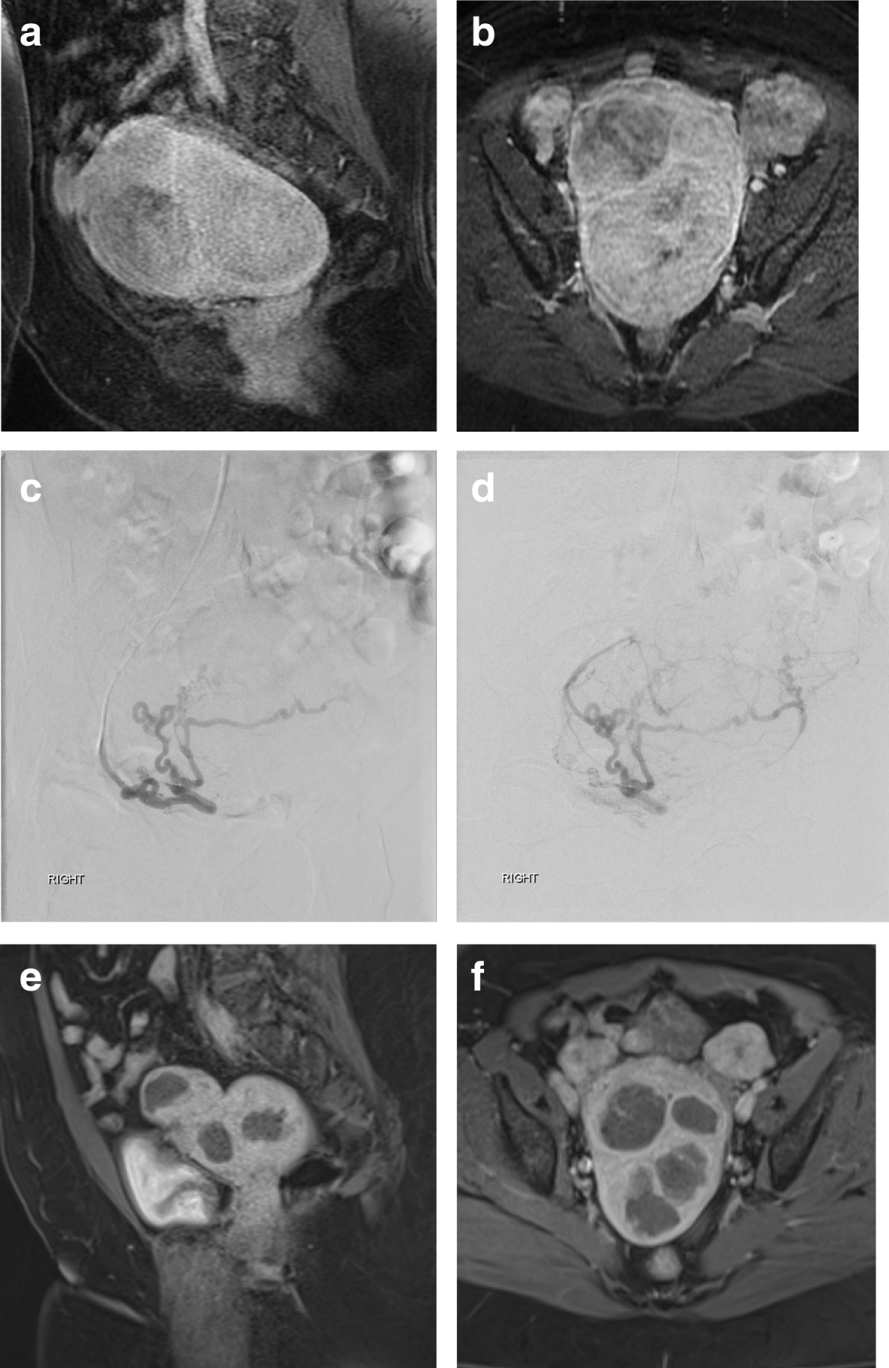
a-f Patient 12 is a 54-year old woman with bulk symptoms including pelvic pressure and heaviness, urinary frequency and urgency, and constipation. Sagittal (a) and axial (b) T1 post-contrast MRI images demonstrate a number of large uterine fibroids with internal enhancement. Digital subtraction angiography (c, d) as part of the UAE procedure demonstrates an enlarged, tortuous right uterine artery, with opacification of multiple masses. The left uterine artery was not identified. Sagittal (e) and axial (f) T1 post-contrast MRI images obtained 232 days after UAE demonstrates near complete necrosis of the majority of the uterine fibroids with decreased size and lack of internal enhancement. The patient had resolution of symptoms
